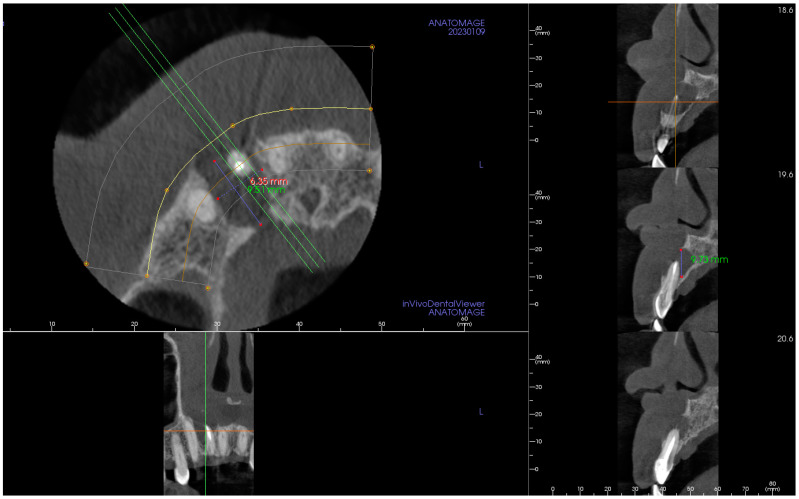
Cone-Beam Computed Tomography showing a three-dimensional image of the lesion, measuring 9.51 × 6.35 × 9.73 mm, on Panorex, Cross-Section, and Paraxial cuts, in correspondence with the upper right lateral incisor, after root canal treatment.
